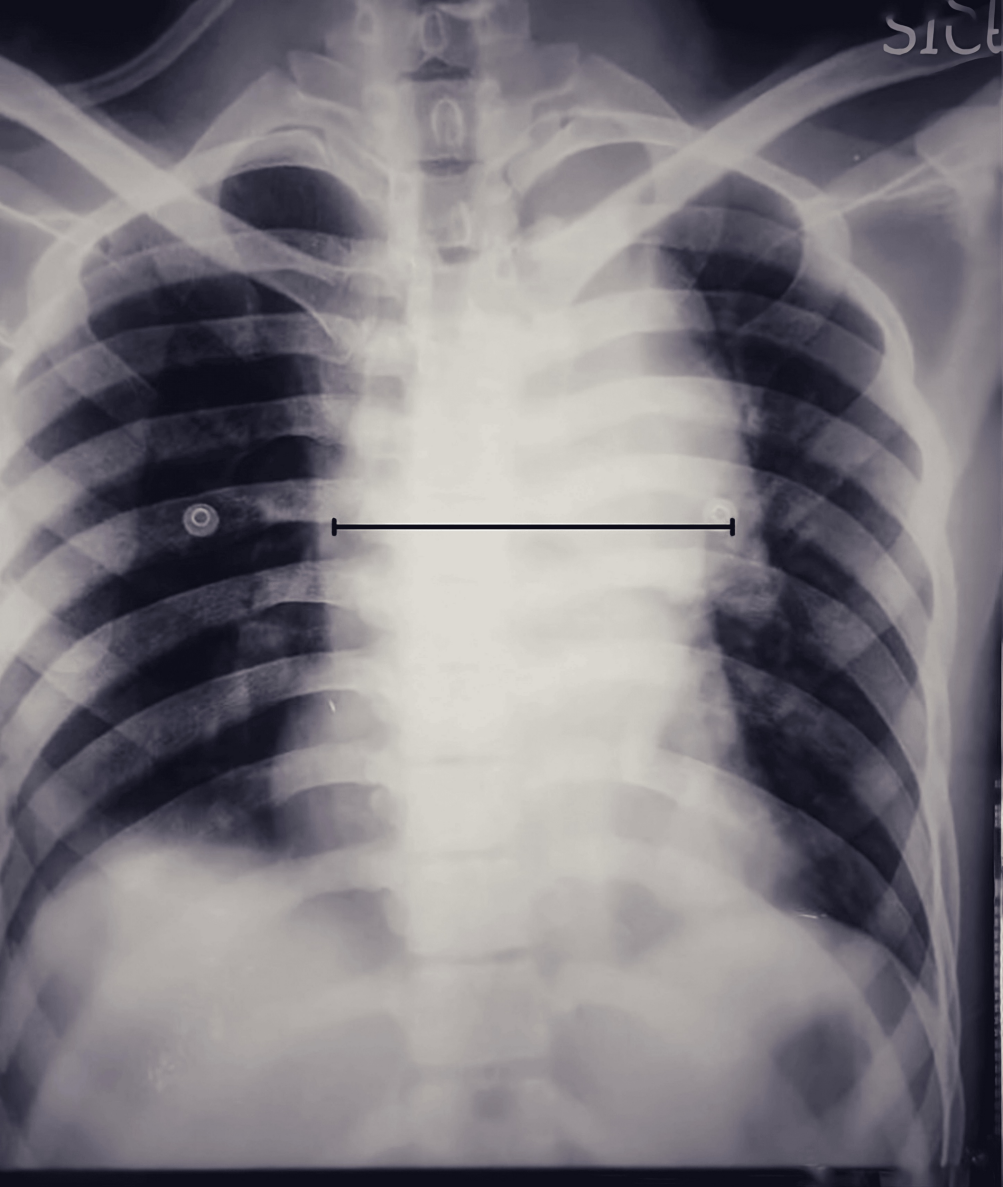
Chest radiograph, in posterior-anterior view showing widening of mediastinum due to aortic aneurysm.
